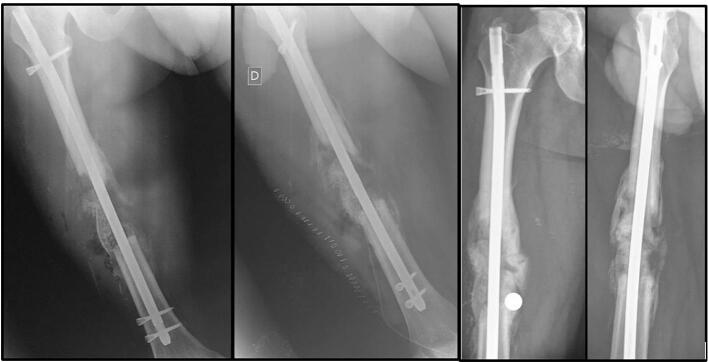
Second stage of modified-Masquelet technique. The cement spacer has been removed and replaced by autologous graft and synthetic bone substitute. No complementary treatment was made to the medial part of the defect. Lateral and antero-posterior X-rays of the femur immediately after surgery (left) and 16 weeks after surgery (right).
